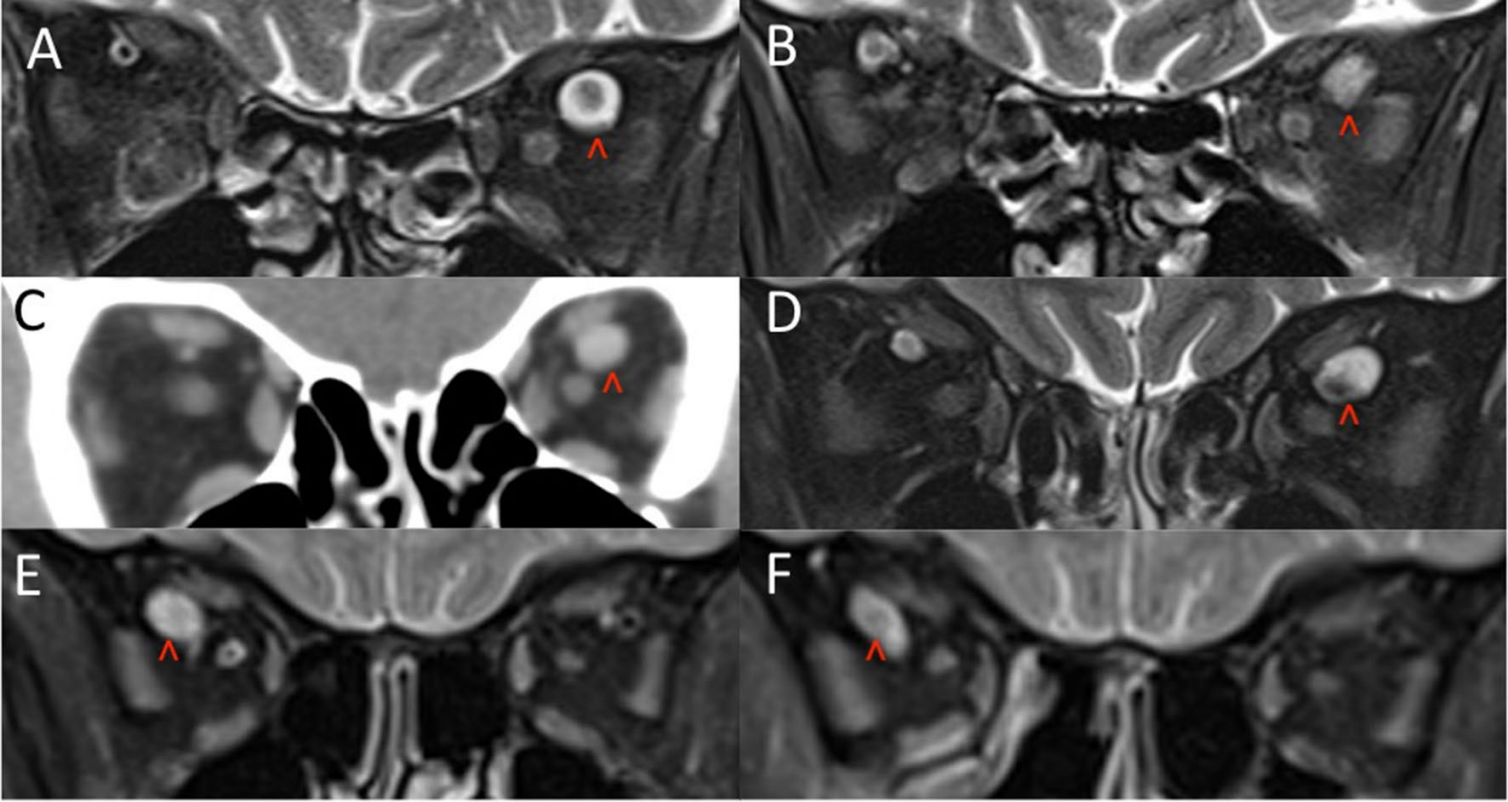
Initial (A) and follow up (B) coronal T2 fat suppressed scans in case 2 showing a left dilated SOV. Initial CT (C) and follow up coronal MRI (D) showing a left dilated SOV in case 3. Case 4 shows a right dilated SOV on initial (E) and follow up (F) scans
